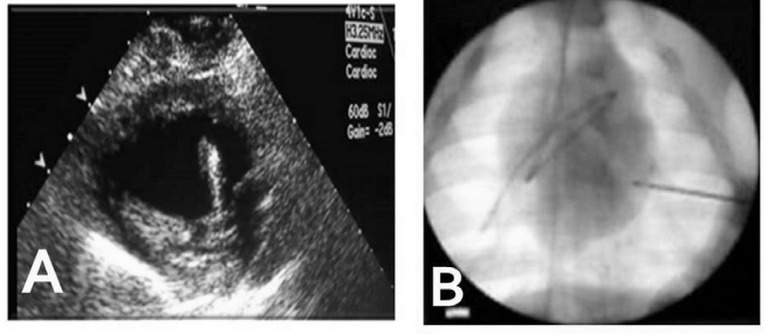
The instrument is easily visualized. A – Echocardiography;
